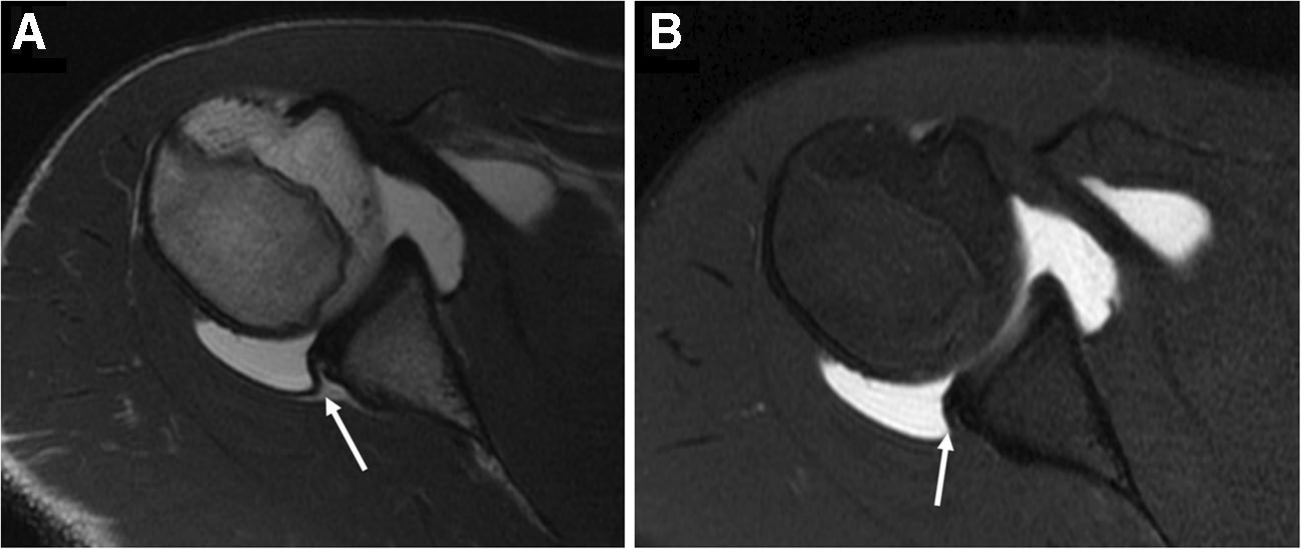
Thrower’s shoulder. Fifteen-year-old baseball player with persistent right shoulder pain. Axial intermediate-weighted (A) and T1-weighted fat-suppressed (B) MR arthrogram images show a thickened posterior band of the inferior glenohumeral ligament at the labral insertion (arrows) with associated glenoid remodeling and retroversion. Constellation of imaging findings was consistent with the clinical diagnosis of glenohumeral internal rotation deficit (GIRD), and the patient was treated conservatively with physical therapy
